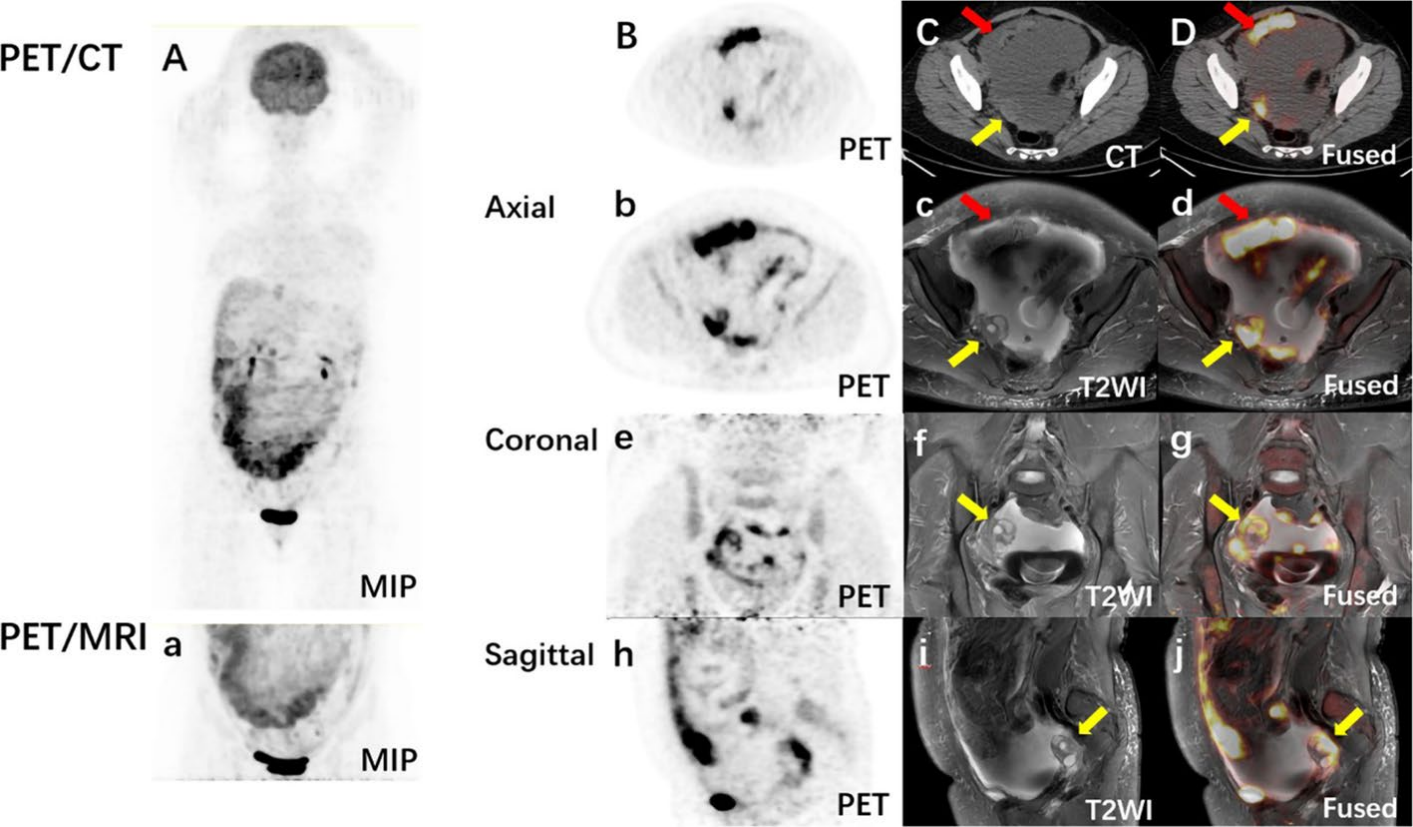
A 47-y-old woman with cancer cells detected in ascites and serum tumor marker CA125 greater than 5000U/ml. PET/CT images (A–D) show two focal increased FDG uptake in the pelvic cavity and corresponding slightly high dense lesions on the CT images (C, arrows). PET/MRI images (a–j) show higher FDG uptake. One lesion was solid (c, red arrow) and the other was cystic solid (c, f, I, yellow arrow) on T2-weighted images. The location and composition of the lesions showed by MRI help distinguish primary ovarian lesions (yellow arrow) from peritoneal metastases (red arrow)
